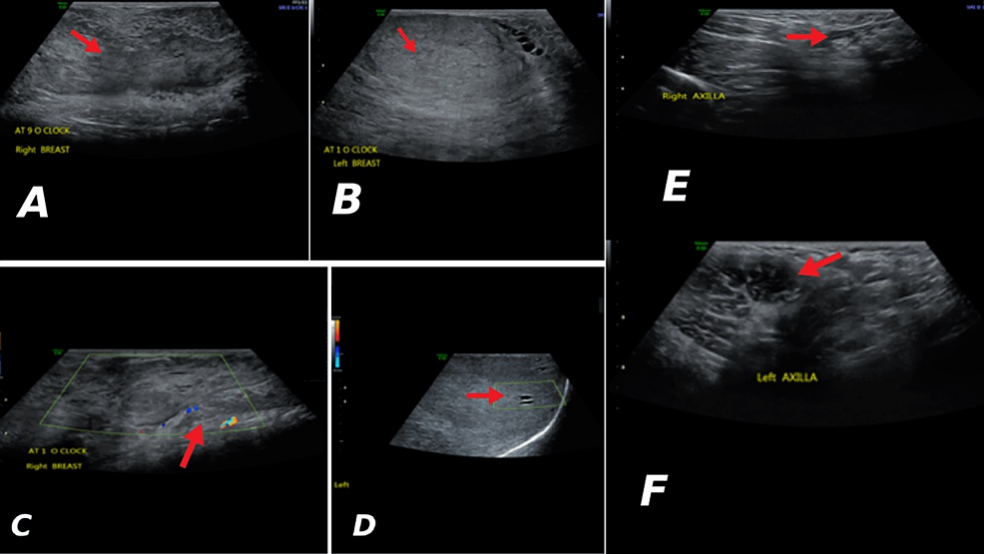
Heterogenous parenchymal echotexture predominantly hyperechoic without definite masses in both right (A) and left (B) breasts and the color doppler images of right (C) and left (D) breasts. No suspicious enlarged axillary lymph nodes in both breasts (E and F).
